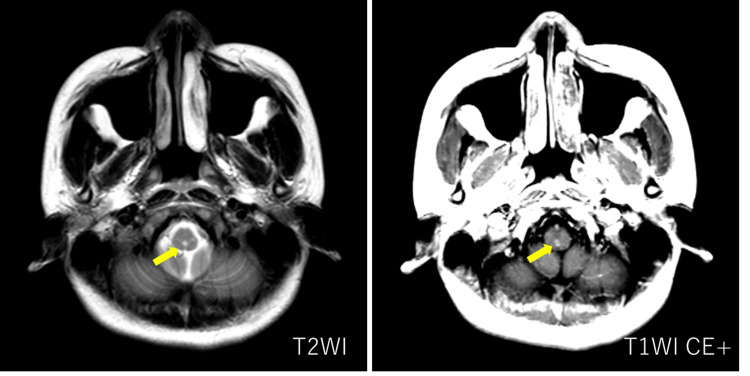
Axial brain MRI at the medullary level Axial T2-weighted MRI showing a hyperintense lesion in the dorsal medulla, with mild enhancement on contrast-enhanced T1-weighted imaging (yellow arrow).
